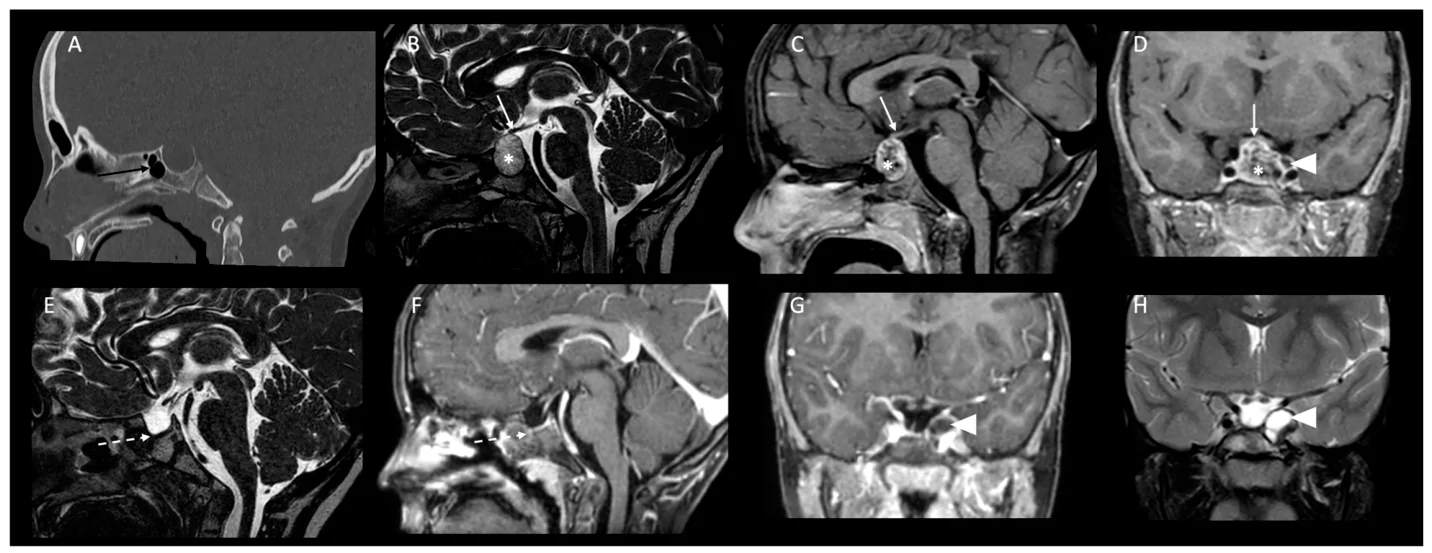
ACP. CT and MRI studies of a 7-year-old boy with headache and worsening vision. (A) Sagittal bone CT, (B,E) sagittal bSSFP, (C,F) sagittal and (D,G) coronal contrast-enhanced T1, and (H) coronal T2. Sella turcica enlargement due to a sellar-suprasellar multiloculated cystic lesion (asterisks), involving the left cavernous sinus (D, arrowhead) and compressing the optic chiasm (white arrows) upward. Note the pneumatization of the sphenoid sinus, best seen on CT (black arrow). Postoperative MRI (E–H) revealed a transsphenoidal approach (dashed arrow), complete ablation of the sellar–suprasellar lesion, and residual involvement of the left cavernous sinus by a cystic lesion with peripheral enhancement (G,H, arrowheads).
